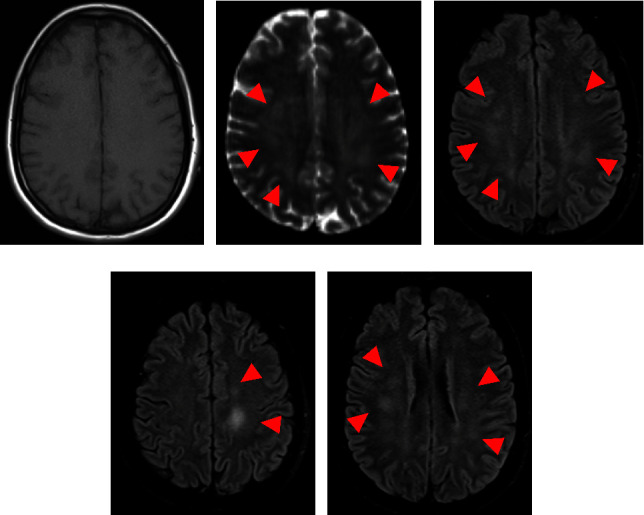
Axial MRI images of the brain at different levels of the brain. (a) T1 image is unremarkable. (b) T2 image and (c) FLAIR image at the same level show multiple foci of abnormal increased signal (red arrow). (d, e) FLAIR images at different levels of the brain demonstrate multiple abnormal foci (red arrow).
